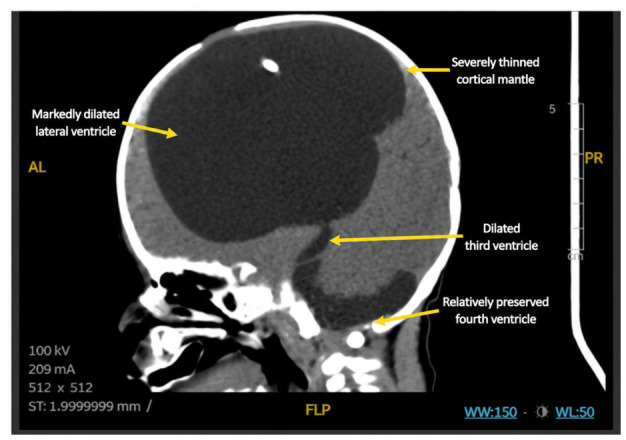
Sagittal CT reconstruction demonstrating marked enlargement of the lateral and third ventricles with relative preservation of the fourth ventricle (arrows), producing an imaging pattern suggestive—but not diagnostic—of a non-communicating hydrocephalus pattern.
